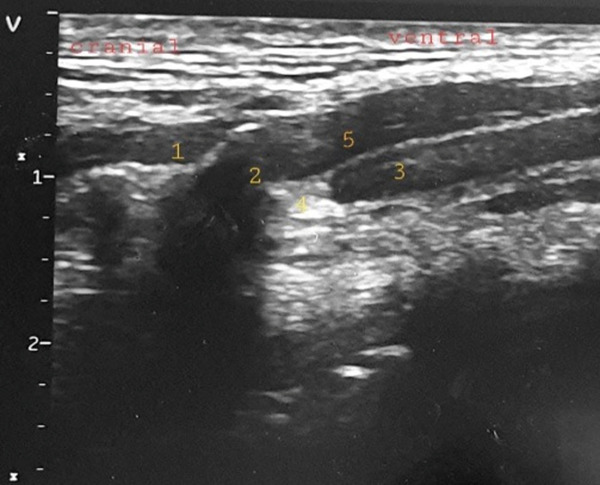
Sonography of left sub-hyoid region 1 – hyoid bone, 2 – thyrohyoid membrane, 3 – thyroid cartilage, 4 – internal branch of the superior laryngeal nerve, 5 – thyrohyoid muscle)
